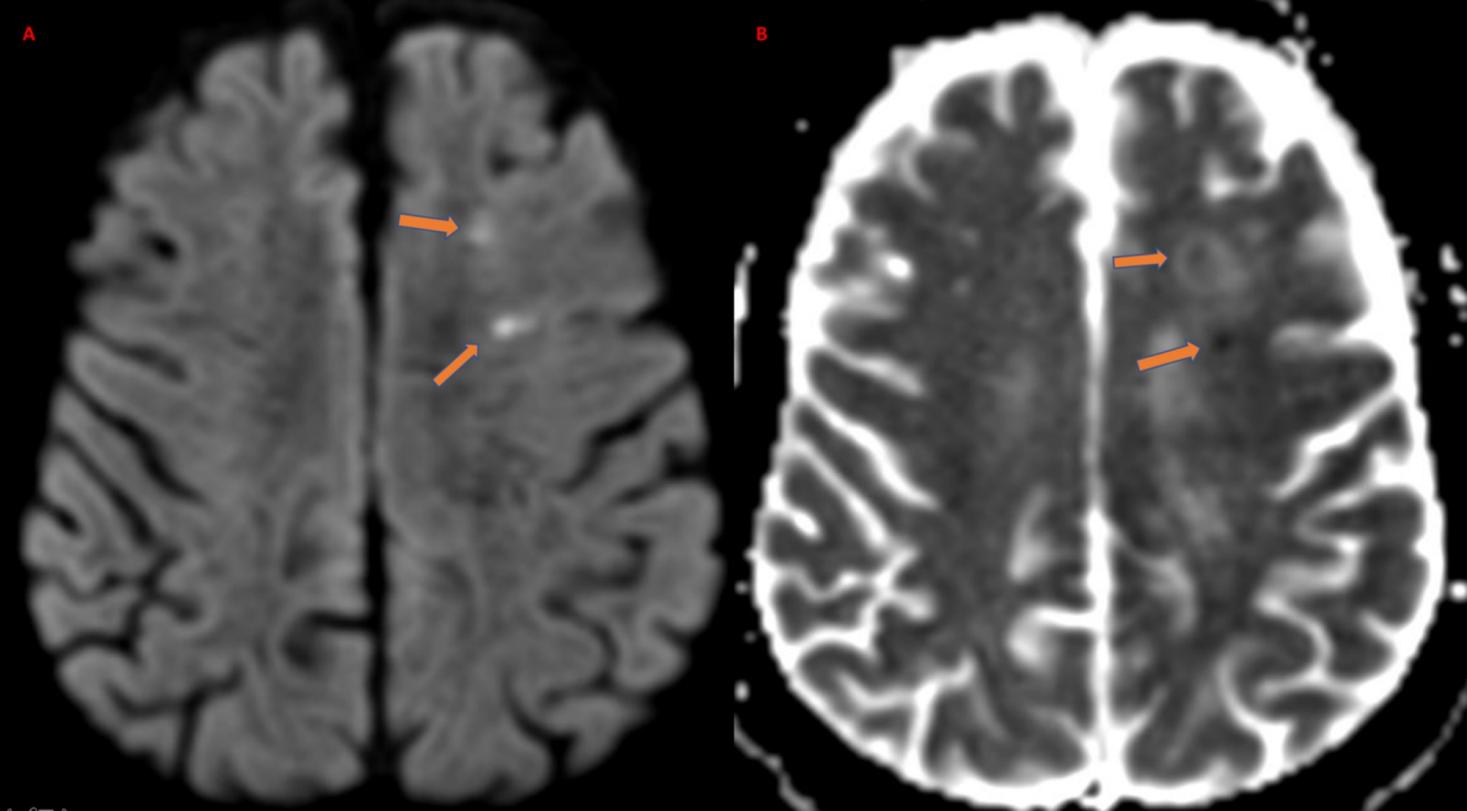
Magnetic resonance imaging of the brain Image A - Diffusion-weighted magnetic resonance imaging (DWI) sequence showing acute infarction in the left frontal subcortical region (orange arrows). Image B - the corresponding apparent diffusion coefficient (ADC) image showing diffusion restriction (orange arrows).
